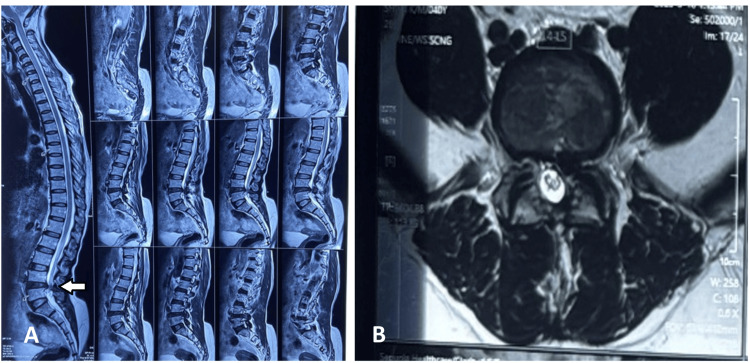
MRI lumbar spine showing prolapsed intervertebral disc at L4–L5 level (A) Sagittal T2-weighted magnetic resonance imaging of the lumbosacral spine demonstrating a posterior disc protrusion at the L4–L5 intervertebral level, indenting the thecal sac and causing anterior compression. Reduced disc height and degenerative signal changes are also noted at the affected level. (B) Axial T2-weighted image at the L4–L5 level showing a left paracentral disc bulge/protrusion causing compression of the traversing left L5 nerve root within the lateral recess, with associated narrowing of the spinal canal.
